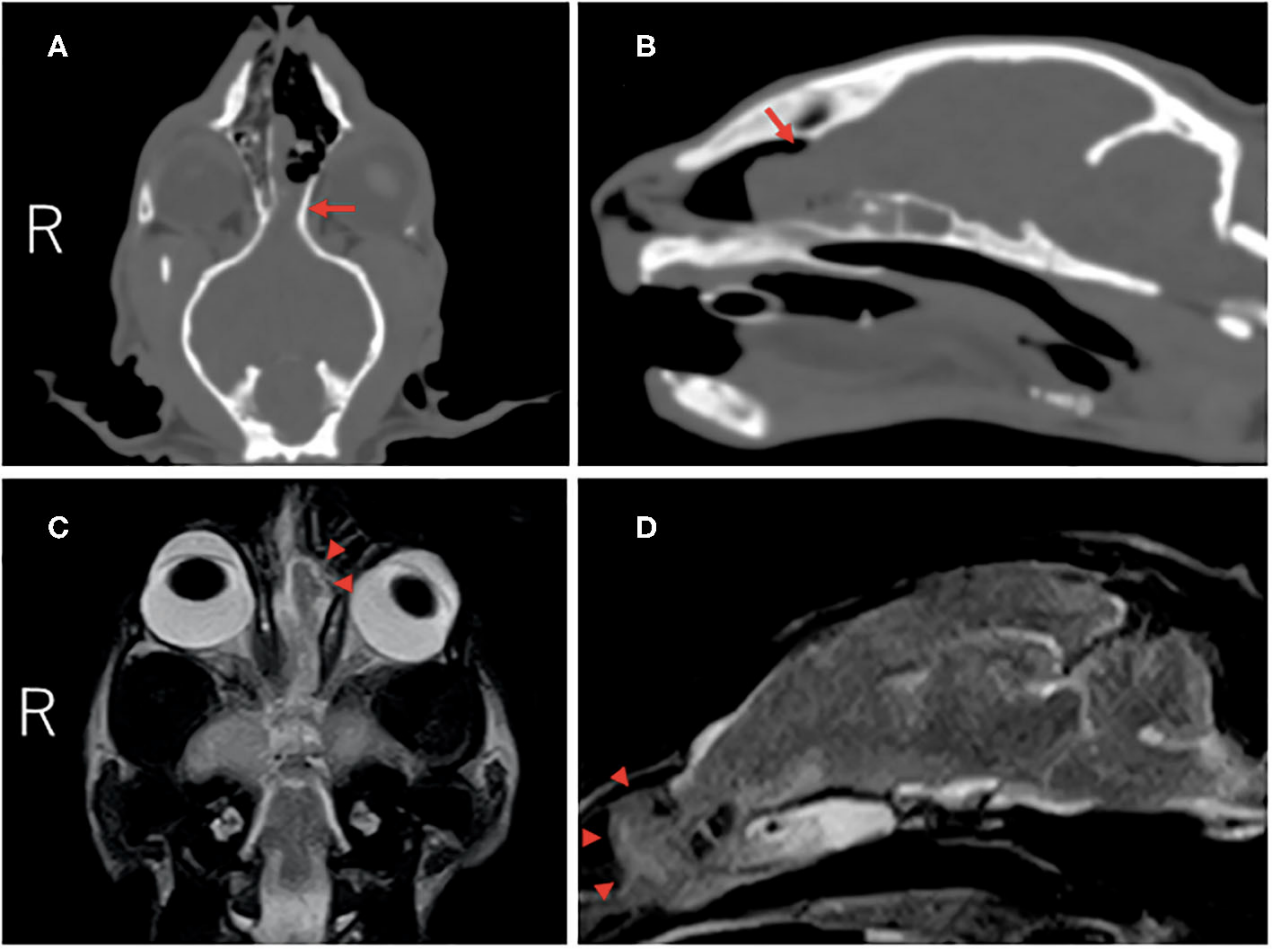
CT and MR images of the cranium and brain at diagnosis. Dorsal (A) and parasagittal (B) CT images showed the defect of the left cribriform plate (red arrow). On dorsal (C) and parasagittal (D) T2-weighted MR images, the left olfactory bulb protruded into the nasal cavity through the defect (red arrowheads).
